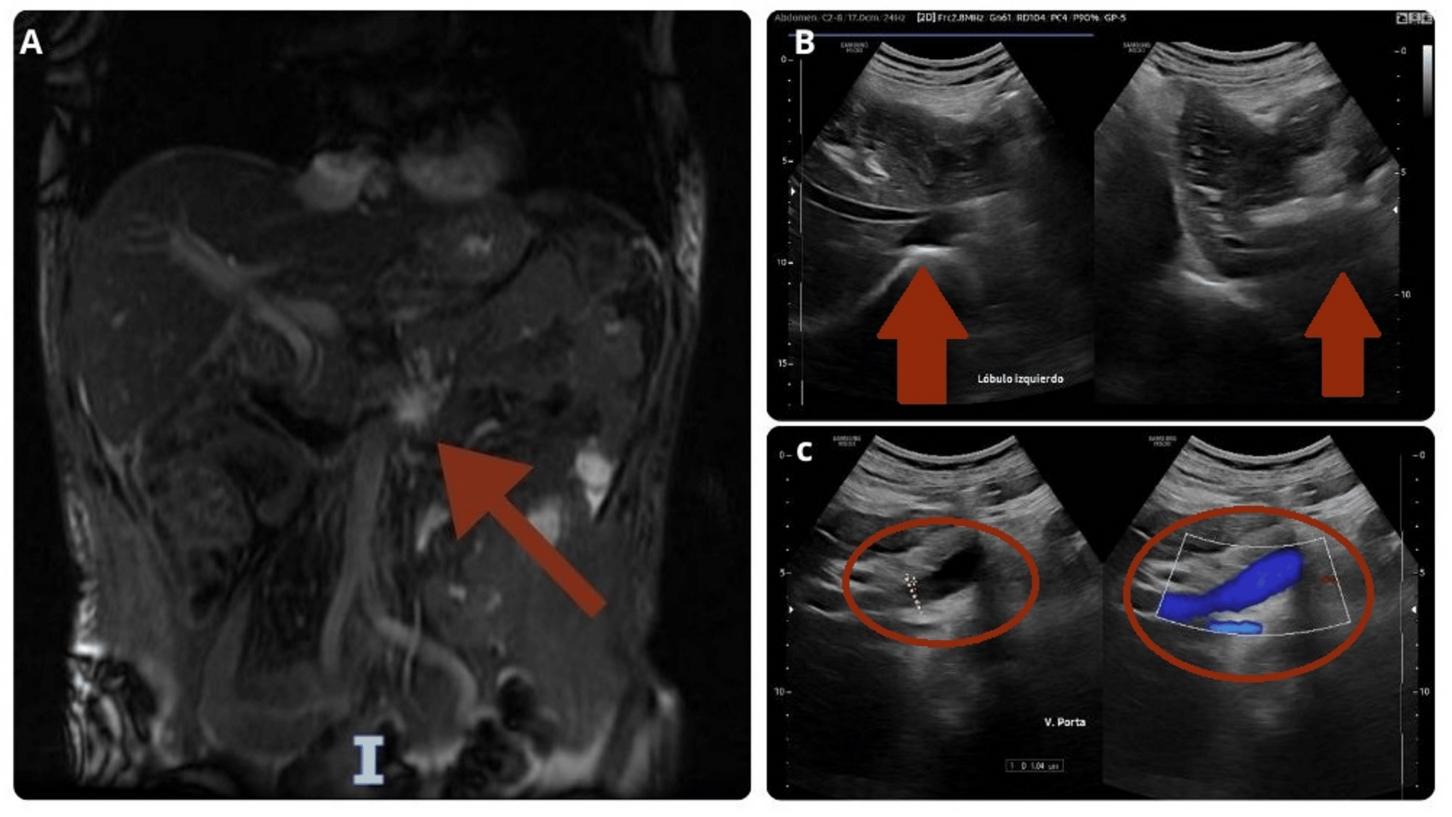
Liver ultrasound and magnetic resonance cholangiography A. Magnetic resonance cholangiography: The liver has a longitudinal diameter of 130 mm, with regular margins and no alterations in the intrahepatic biliary tract. The common bile duct measures 7 mm in diameter. B. Left lobe: A heterogeneous pattern is observed. C. Portal vein measures 10 mm. No anatomical abnormalities detected.
